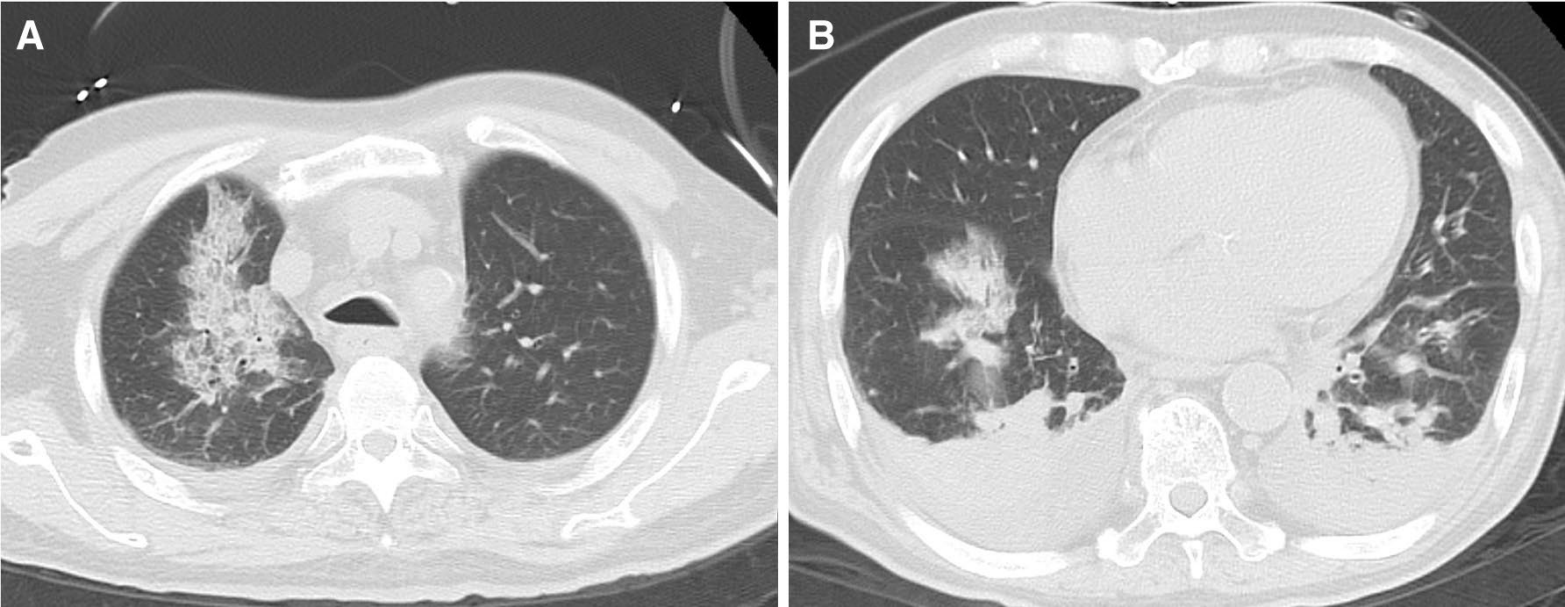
Pulmonary CT on the day before admission. a, b Bilateral multiple consolidation and ground-glass opacity. b Bilateral pleural effusion was seen
